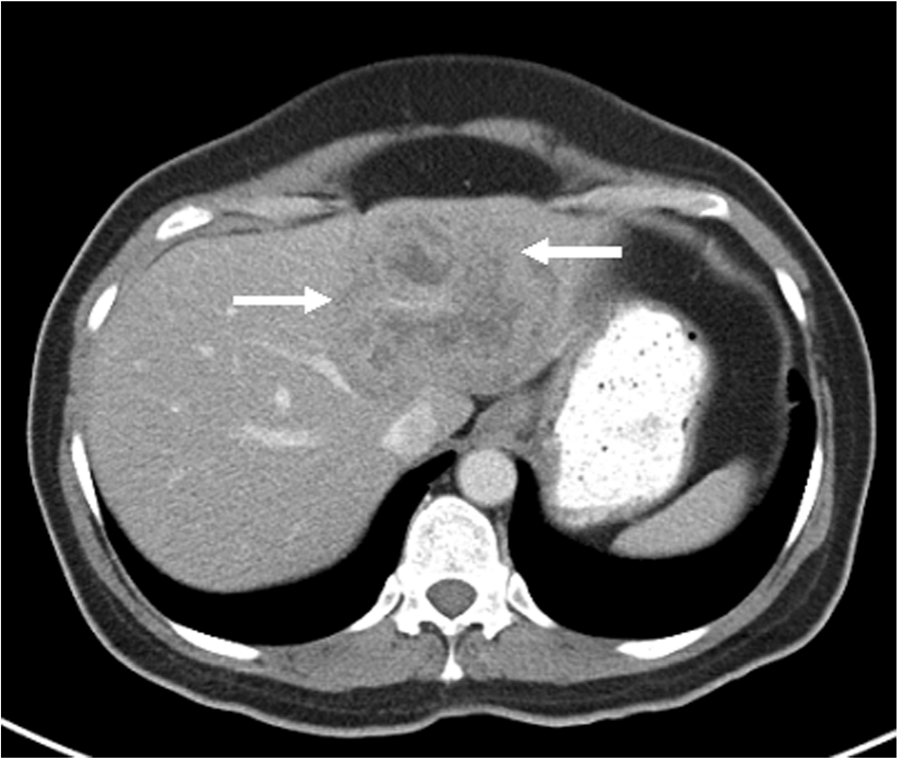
Pre-treatment CT scan of the abdomen showing a large hypodense mass in the left lobe of the liver (marked by arrows); biopsy of the mass revealed metastatic HER2-positive breast cancer.
